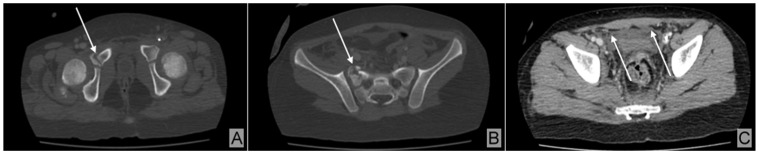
Pelvic CT scan findings. (A) Fractures of the pubic ramus and right sacral wing (arrow). (B) Displaced fracture of the right sacral wing (S1) (arrow), along with a fracture and displacement of the outer third of the right ilio-pubic ramus. (C) Hyperintense signal in the pelvic region adjacent to the fracture, indicating pelvic fat infiltration (arrows) due to the impact.
